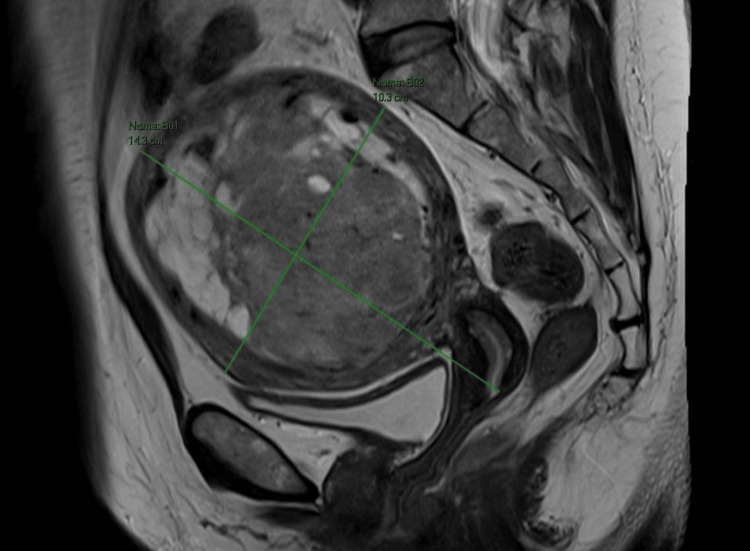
MRI pelvis T2 sagittal view showed enlarged heterogenous fibroid prominent nonenhancing cystic spaces
